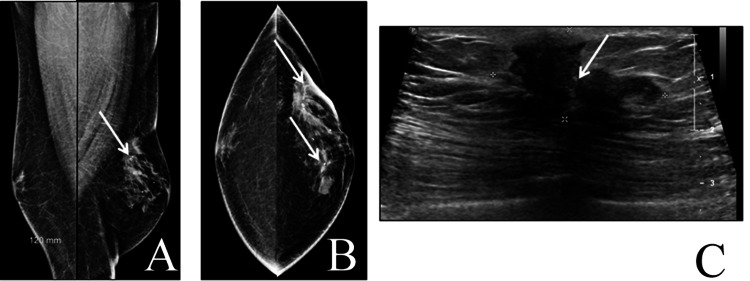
A 60-year-old male with left breast swelling of three years' duration. Mammograms revealed multicentric spiculate masses at the left upper outer quadrant with skin retraction and lower inner quadrant (arrowed) considered BI-RADS 5 and also confirmed in ultrasound. Staging revealed axillary, lung, and bone metastases. Biopsy as well as modified radical mastectomy confirmed multicentric lesions, invasive ductal carcinoma, hormone receptor-positive and human epidermal growth factor receptor 2 (HER2) negative. (A) Bilateral mediolateral oblique mammograms. (B) Bilateral craniocaudal mammograms. (C) Corresponding ultrasound of the left upper quadrant.
